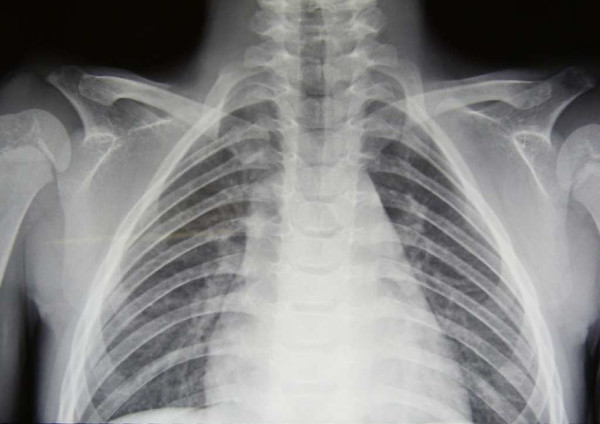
Chest X-ray showing bilateral clavicular hypoplasia.
